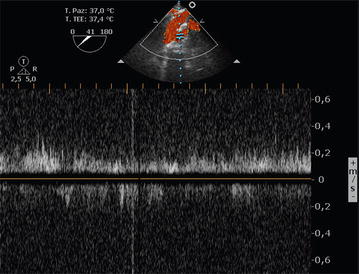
Doppler analysis of the blood flow through the cavo-cavostomy rescue anastomosis, showing appropriate liver venous drainage
